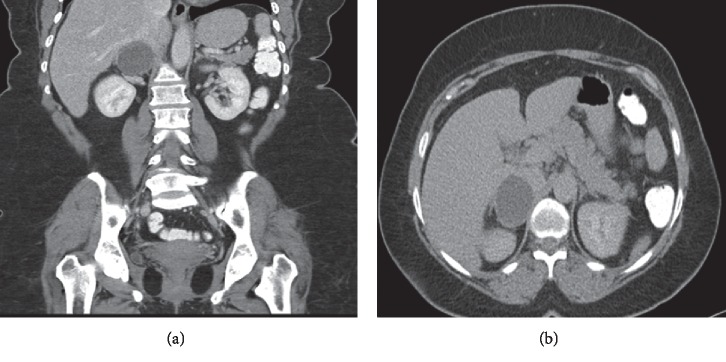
Preop CT adrenal: (a) coronal section and (b) axial section showed a 4.8 × 4.1 cm well-defined rounded right adrenal hypodense lesion. It has precontrast density of 12 HU. Its density measured 10 HU in the porto-venous phase and 15 HU on the delayed 15 minutes phase. No wall calcification. The left adrenal gland is within normal.
